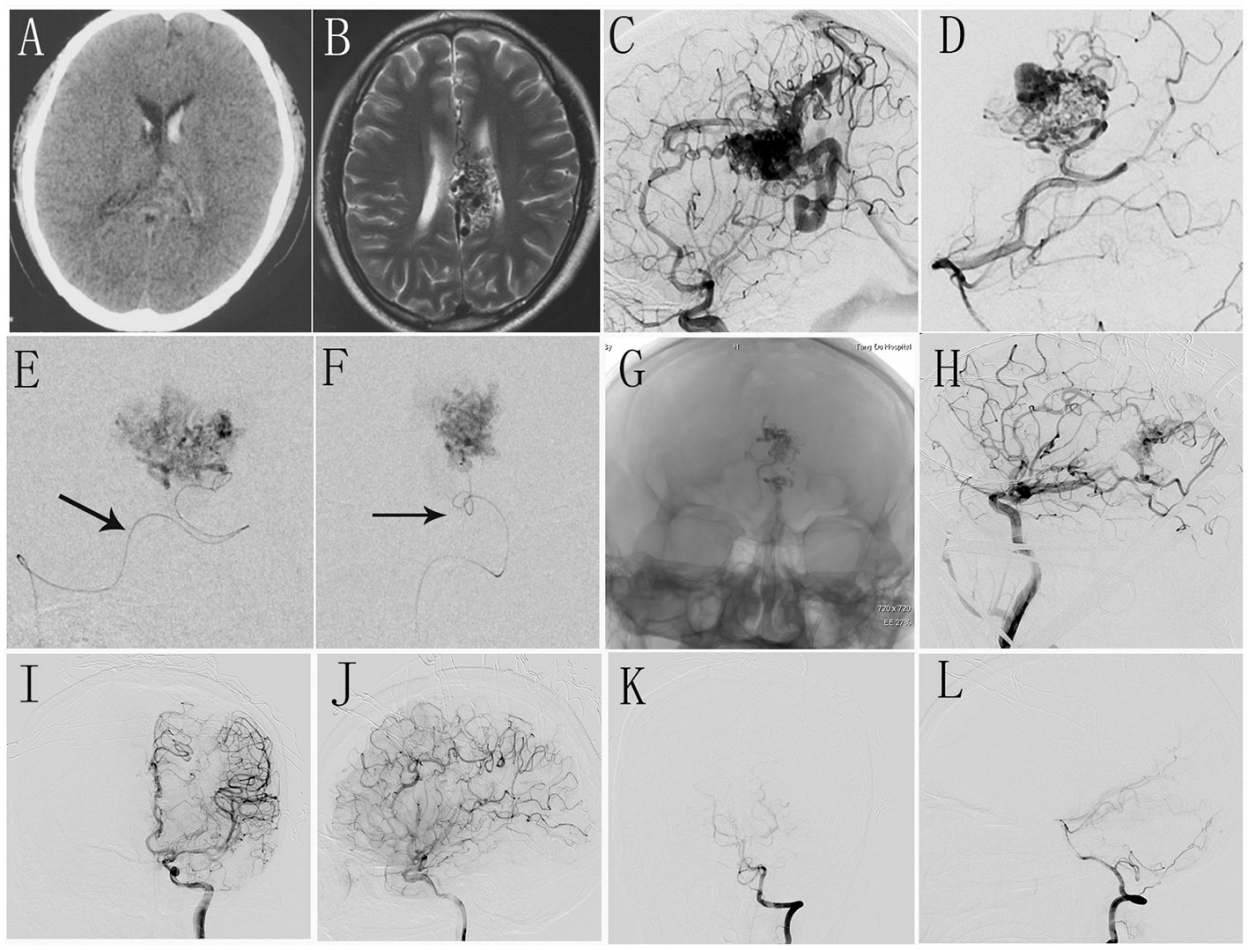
(A,B) Preoperative CT and MR scan shows intraventricular hemorrhage and malformed vascular mass in corpus callosum. (C,D) Intraoperative DSA shows AVM located in corpus callosum with dual blood supply from anterior cerebral artery and posterior cerebral artery. (E,F) Selective angiography shows the deep feeder artery and embolization was performed. (G) The morphology of Onyx glue under X-ray view. (H) Intraoperative DSA shows the residual AVM nidus. (I–L) The immediate postoperative DSA shows complete occlusion of AVM.
